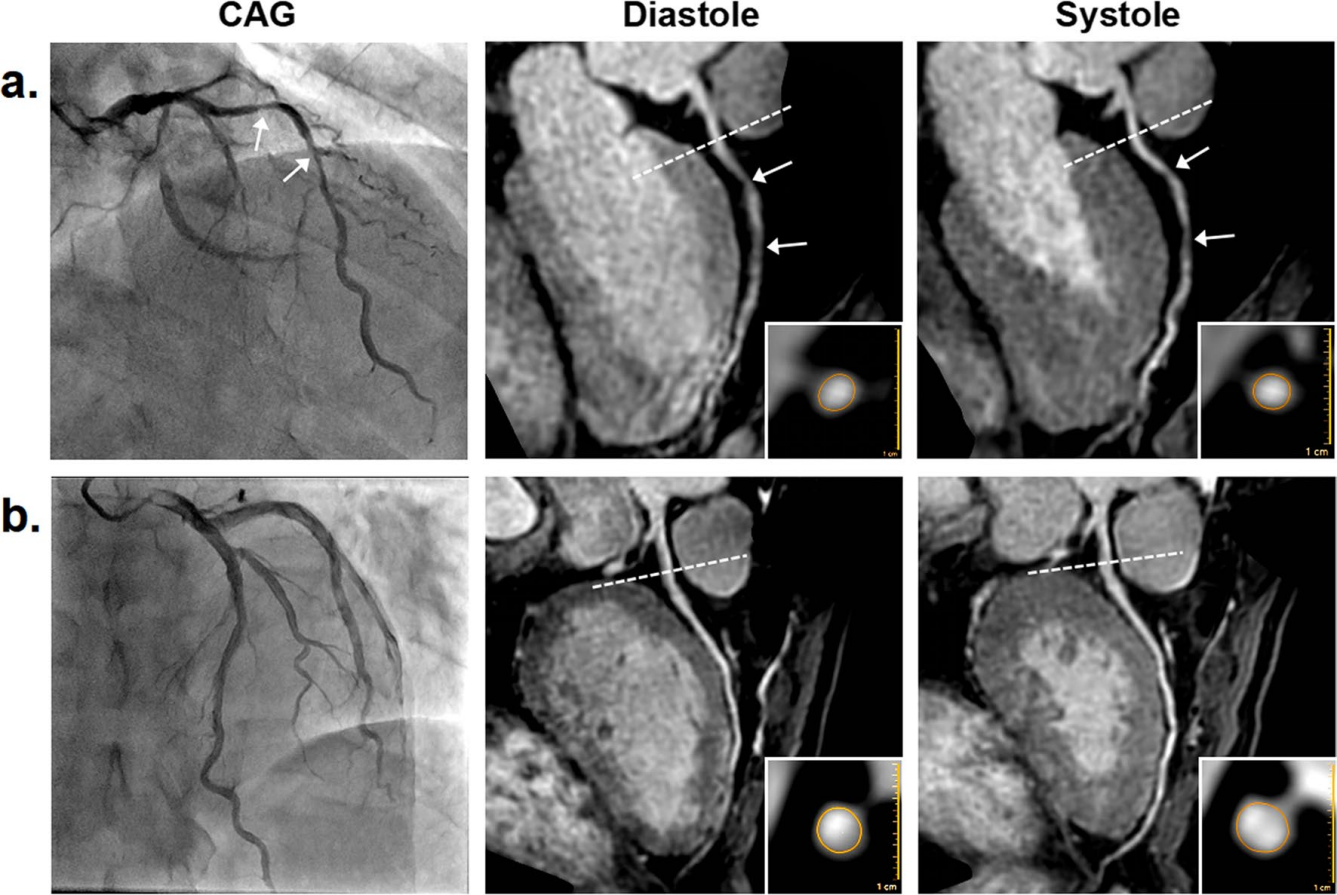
Coronary distensibility of two suspected coronary artery disease (CAD) patients measured with coronary MR angiography (MRA). a Images in a 55-year-old male suspected CAD patients. Peripheral blood pressure was 140/92 mm Hg (pulse pressure = 48 mm Hg). The X-ray coronary angiography (CAG) image shows significant stenoses in the proximal and middle left anterior descending artery (LAD). Coronary MRA images show slight coronary distensibility in LAD: lumen area 7.28 mm^2^ at diastole and 7.89 mm^2^ at systole, coronary distensibility index (CDI) = [(7.89 − 7.28)/(7.28/48)] × 1000 = 1.75 mm Hg^−1^. b Images in a 63-year-old male suspected CAD patients. Peripheral blood pressure was 134/90 mm Hg (pulse pressure = 44 mm Hg). CAG shows a normal LAD without significant stenosis. Coronary MRA images show moderate coronary distensibility in LAD: lumen area 11.79 mm^2^ at diastole and 14.60 mm^2^ at systole, CDI = [(14.60 − 11.79)/(11.79/44)] × 1000 = 5.42 mm Hg^−1^
